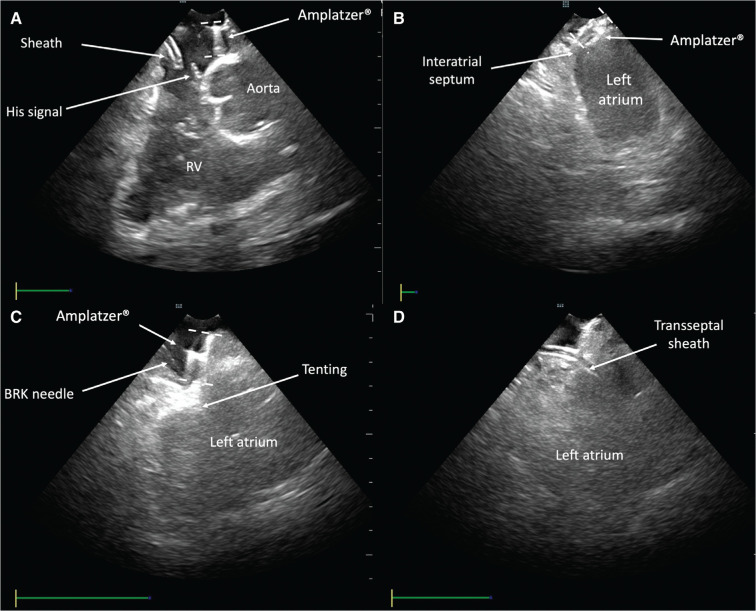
ICE images taken during the procedure. A: Home view showing the Amplatzer® device and the His signal catheter. B: A point in the native septum was selected for left atrium access. C: ICE was used to guide septum puncture. D: Access to the left atrium. ICE: intracardiac echocardiography BRK: Brockenbrough; RV: right ventricle.
